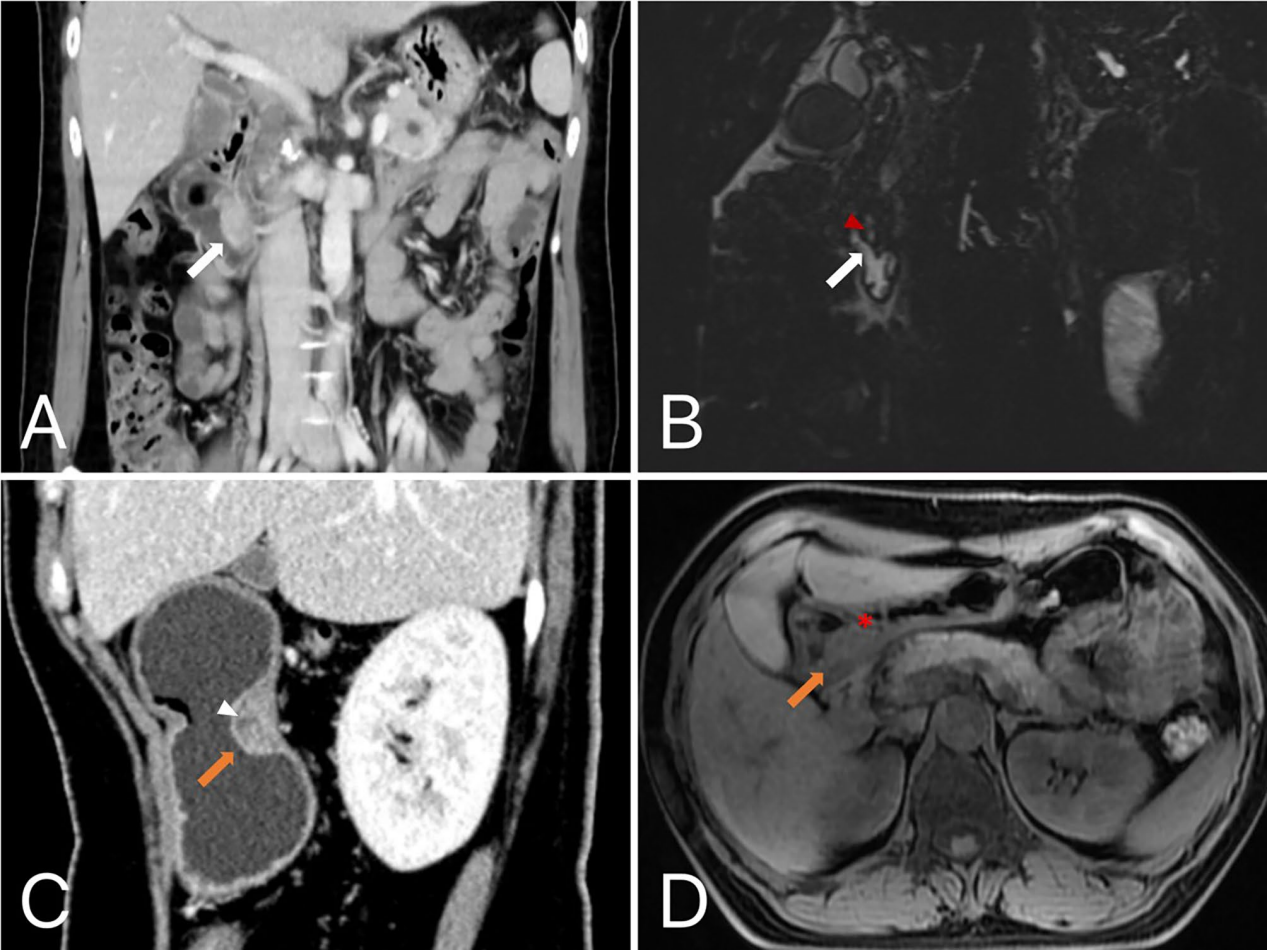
(A-B) A HP in duodenal papilla. The coronal image in portal venous phase showed a 3 cm, ovoid and ill-defined lesion (white arrow) with intraluminal growth pattern, presented marked and heterogeneous enhancement totally. A single-opening duct-like structure that opened into the duodenal lumen was showed in the MRCP image (red arrowhead). (C-D) A HP located in posterior wall of the gastric antrum. The sagittal CT image in arterial phase showed a 2.6 cm, hill-like submucosal lesion (orange arrow) with intraluminal growth pattern, and demonstrated peritumoral hypodensity line, surface ulceration (white arrowhead). The axial T1-weighted MR image showed similarly a broad- based, microlobulated (*) lesion with isointensity
